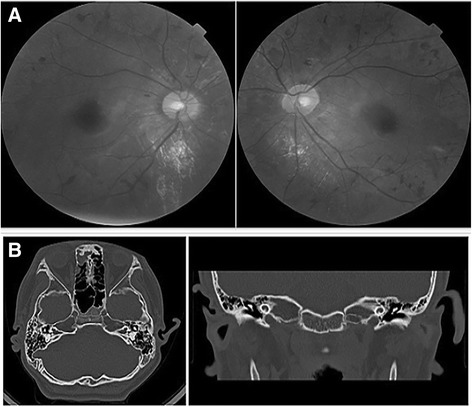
a Ophthalmoscopy photos of the patient showed the partial degeneration of the choroid in both eyes. b High-resolution CT imaging showed the cochlea deformity of the patient, including the short base turn of the cochlea, unclear division between the second turn and the apical turn of the cochlea and the absence of the modiolus
